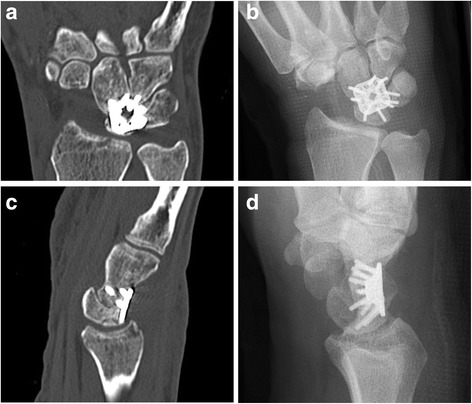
The plate position appears to be correct in the initial review of the X- Ray b: anteroposterior view, d: sagittal view], but the CT scans (a, c) revealed a proximal pate placing
